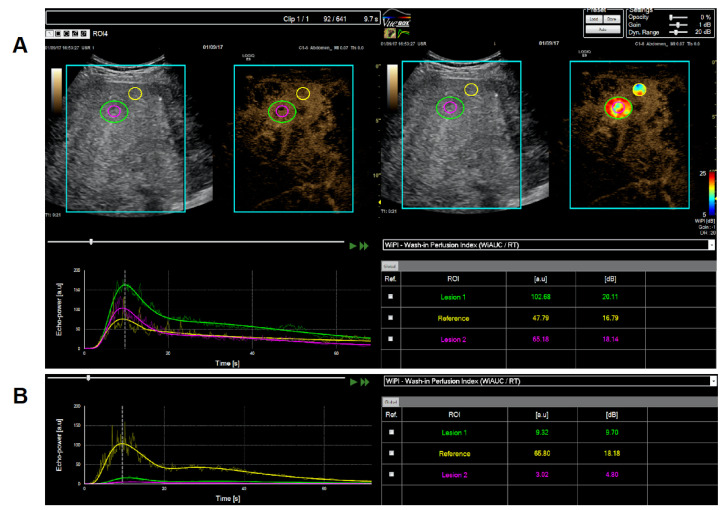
Contrast-enhanced ultrasound (CEUS) measurement (UPN 006) of wash-in perfusion index (WIAUC/RT, see Supplementary Figure S1) in liver metastasis (green and purple) versus normal liver (yellow) reference region of interest (ROI) before (upper panel, (A)) versus after 5 days of treatment with 2.5 mg/m^2^ tTF-NGR per day (lower panel, (B)).
